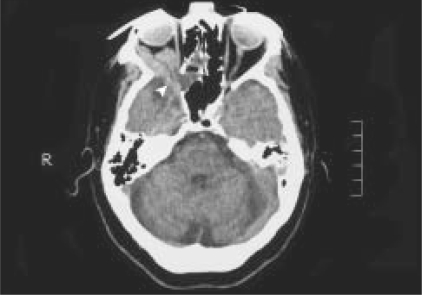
Computed tomographic scan showing absence of the greater wing of the sphenoid on the right side (arrowhead) and a defective right medial wall
